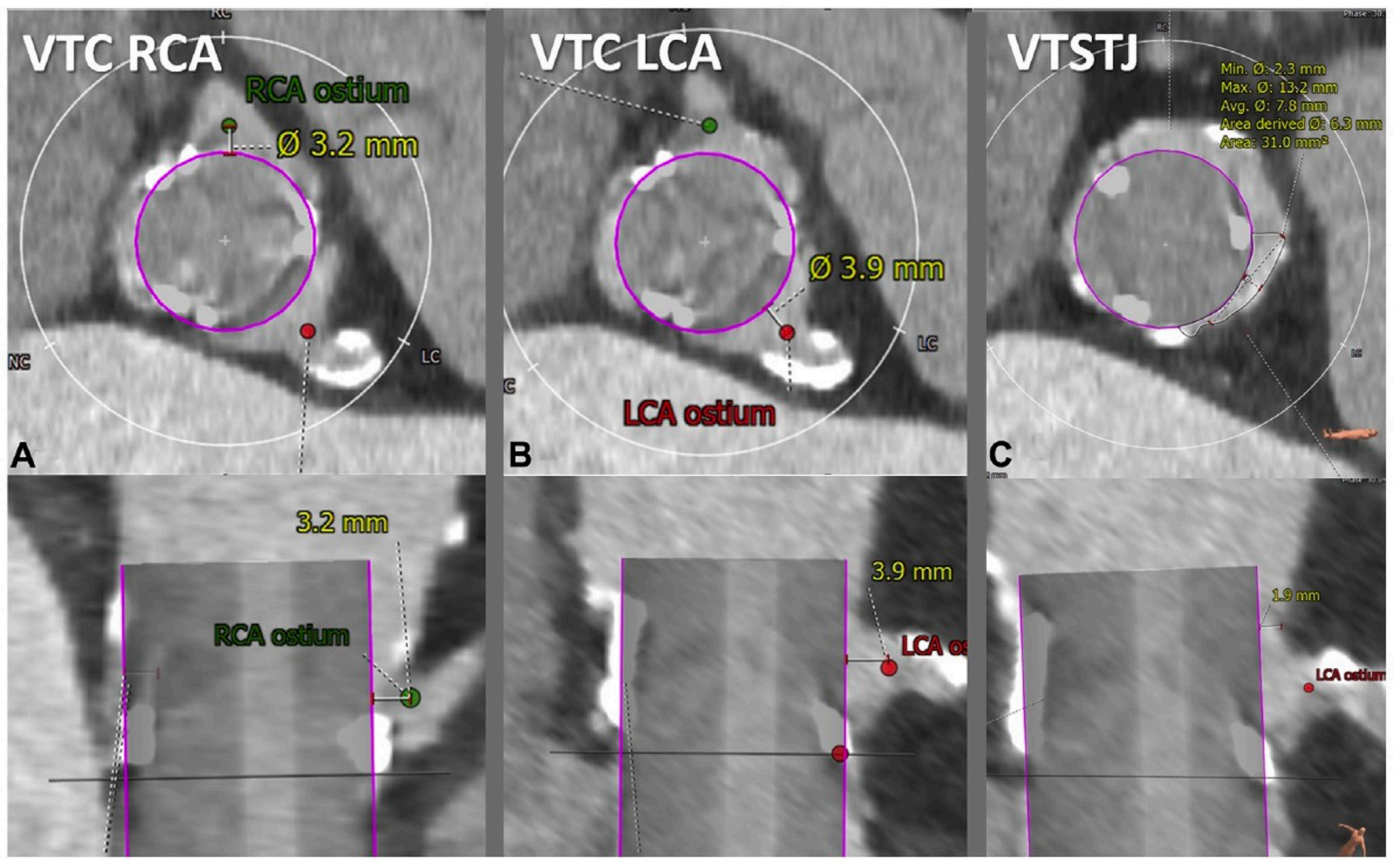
Cardiac computed tomography showing the virtual valve–to–coronary (VTC) distance, which is measured in 2 orthogonal planes (top, axial; bottom, longitudinal). Representative VTC distance measurement for (A) a right coronary artery (RCA) and (B) left coronary artery (LCA). The valve-to-sinotubular junction (VTSTJ) distance is measured in orthogonal planes (C): axial (upper) and longitudinal (lower), when the sinotubular junction is lower than the height of the transcatheter aortic valve replacement device. Reprinted with permission.^9^
